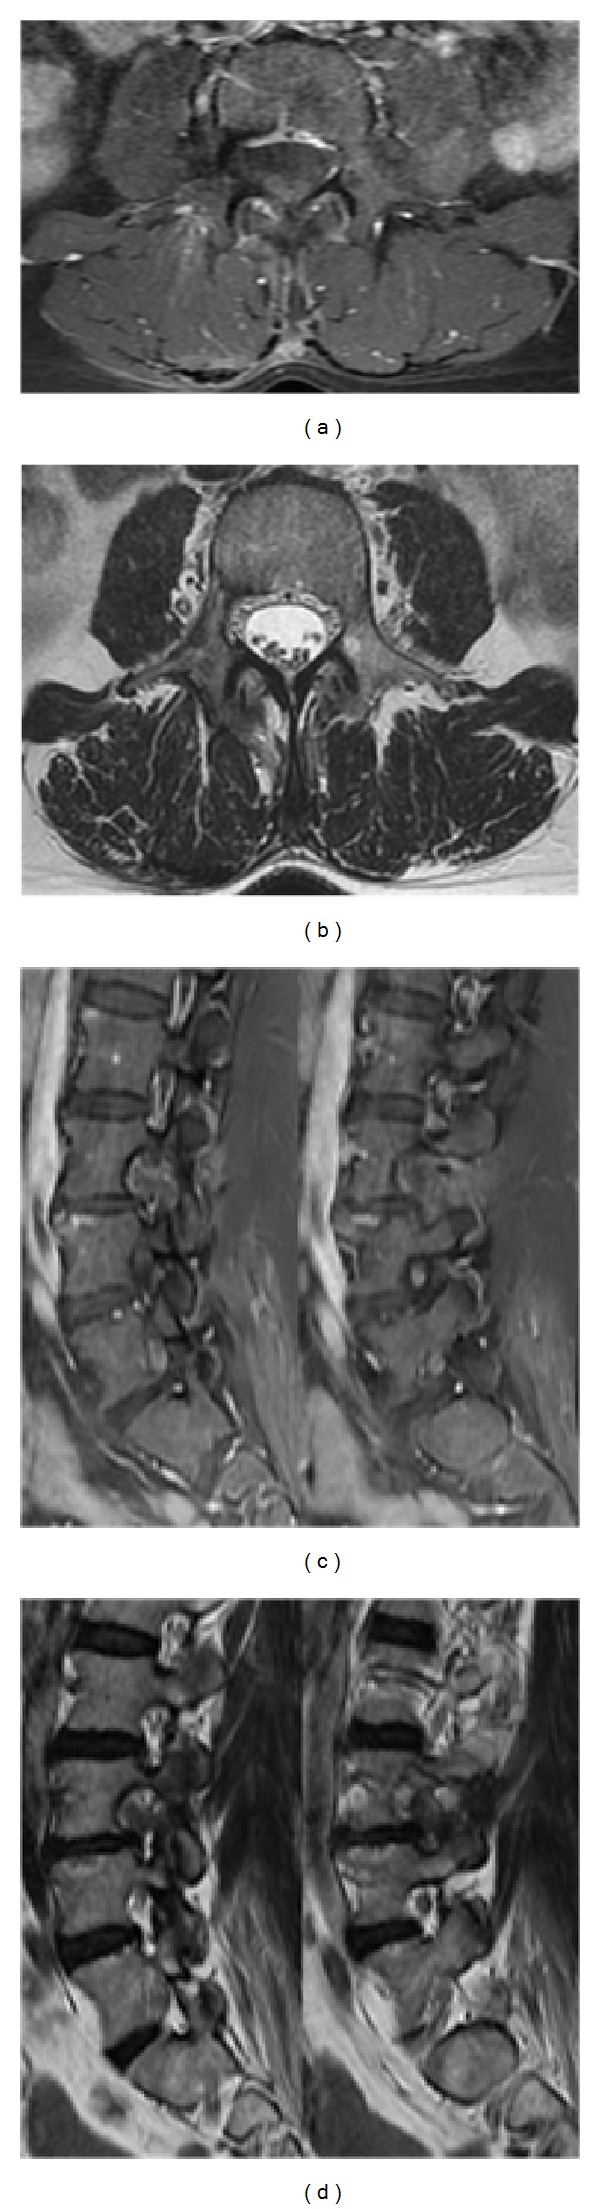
Postoperative MRI demonstrates gross total resection of the tumor and postoperative changes with no muscle atrophy: (a) axial T1-weighted with gadolinium enhancement; (b) T2-weighted; (c) sagittal T1-weighted with gadolinium enhancement; (d) T2-weighted.
